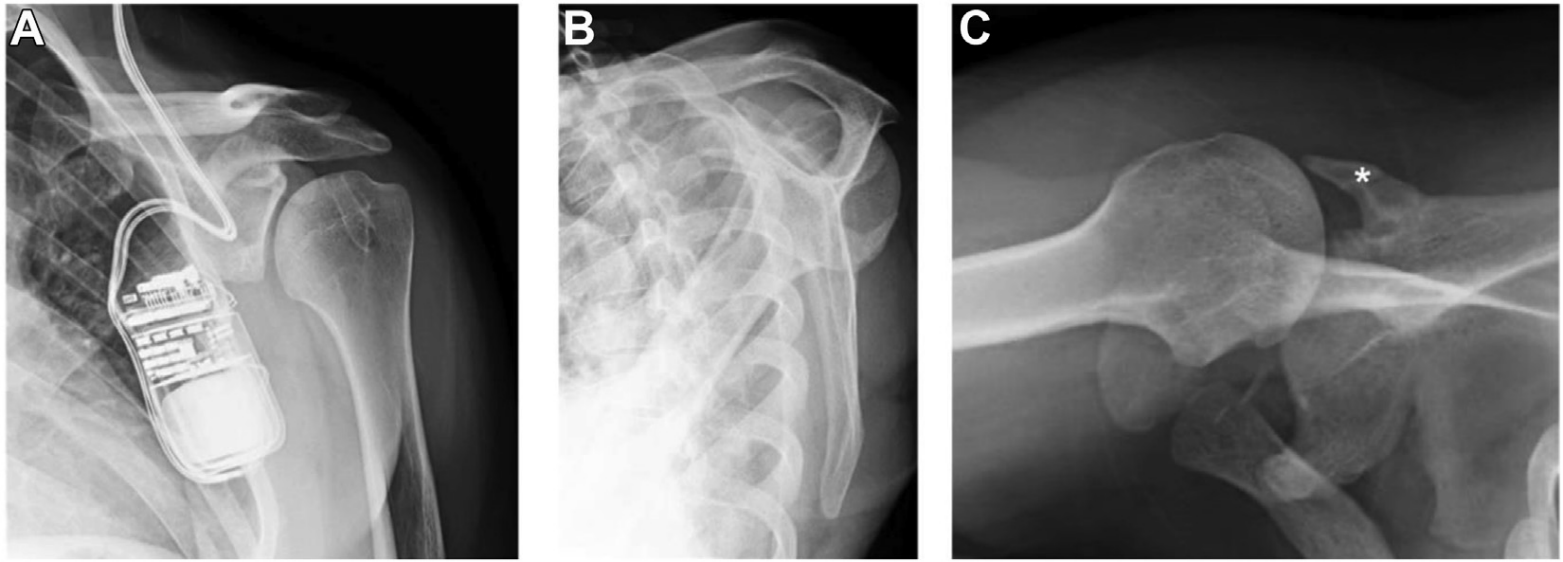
Plain film radiographs of the left shoulder (A) Grashey, (B) scapular Y, and (C) axillary. Significant posterior bone loss of the native glenoid with a posterior glenoid iliac crest bone graft (∗) can be seen. Lucency within the humeral head can be appreciated and resulted from prior procedures, and an implanted neurostimulator, which can be partially visualized.
